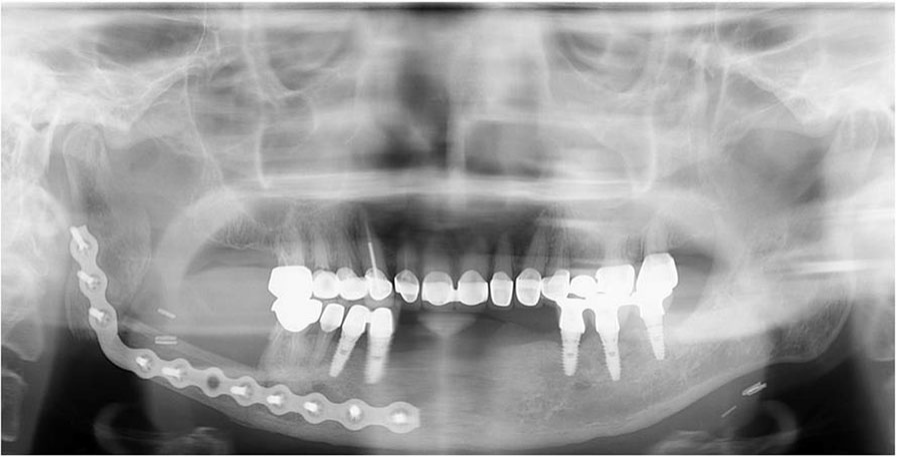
Postoperative panoramic radiograph showing fixation of fibular free flap
